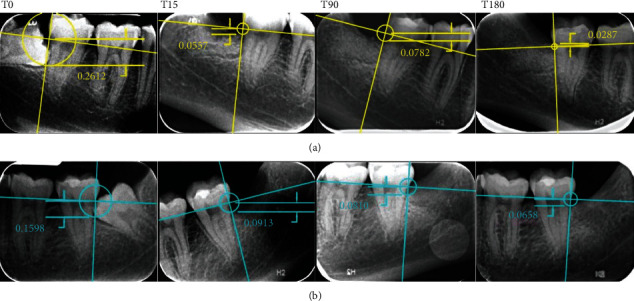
Periapical radiographs of the grafted site (a) and the control site (b) before surgery (T0) and after 15, 90, and 180 days. Since the positioning of the film in a so posterior area resulted difficult, a rigid support held by the patient was adopted instead of the Rinn centrator. Adjustments to tilted images were performed by the same software used for the measurements.
